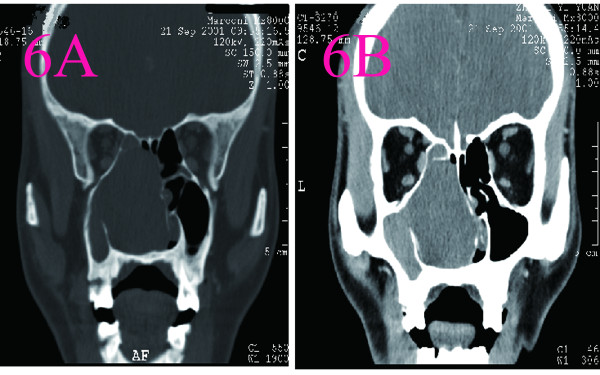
CT scans of nasal cavity in Case 5. A:A well-defined, inhomogeneous, 1.0 cm × 1.0cm soft mass that located at the anterior of the left nasal vestibule was observed by CT scan. The mass had an attenuation number of 17 (Hu). B:There was homogeneous enhancement on contrast CT(50Hu).
